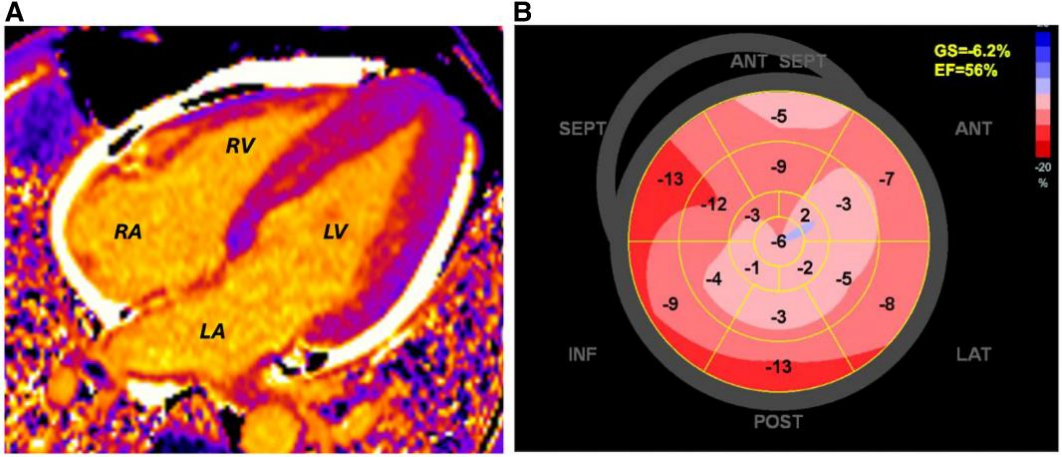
(*A*) Native T1 mapping of 2023 MRI (apical four-chamber view). Purple areas correspond to lower values and orange areas to higher values. The overall T1 time was 1084 ms. (*B*) Longitudinal strain map of 2023 echocardiogram. LV, left ventricle; RV, right ventricle; LA, left atrium; RA, right atrium; GS, global strain; EF, ejection fraction; ANT SEPT, anteroseptal; SEPT, septal; INF, inferior; POST, posterior; LAT, lateral; ANT, anterior.
